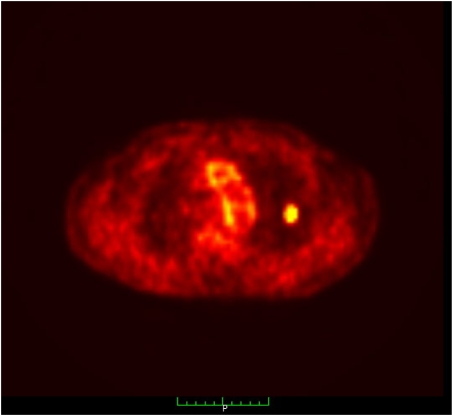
PET/CT image of single lung metastasis for RFA. This is a typical lesion and ideal for RFA.
